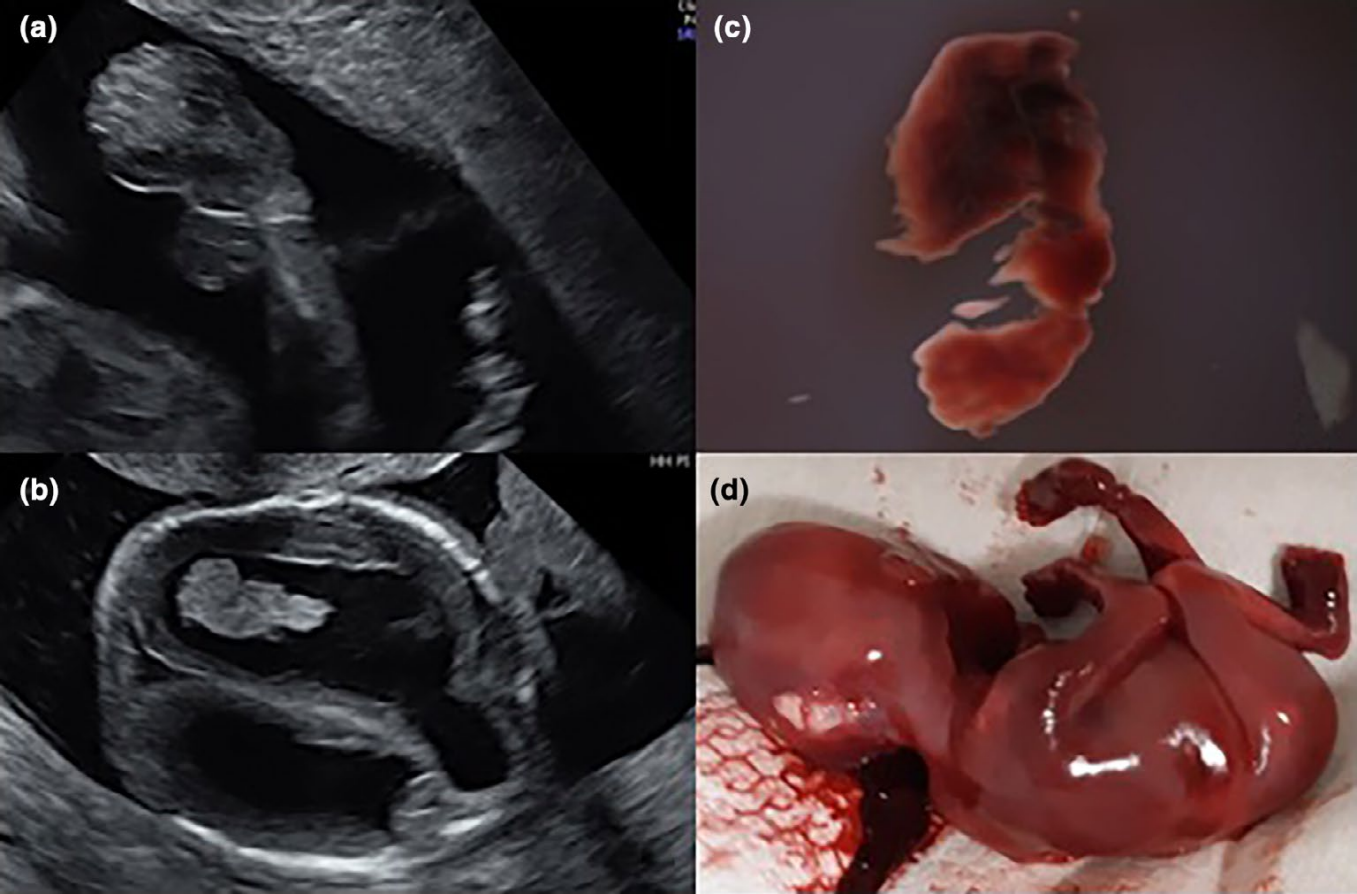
(a, b) Prenatal ultrasound scan in fetus with multiple malformations at 16 gestational weeks. (c, d) Therapeutic interruption due to posterior occipital encephalocele and lower limb defect from amniotic band syndrome.
